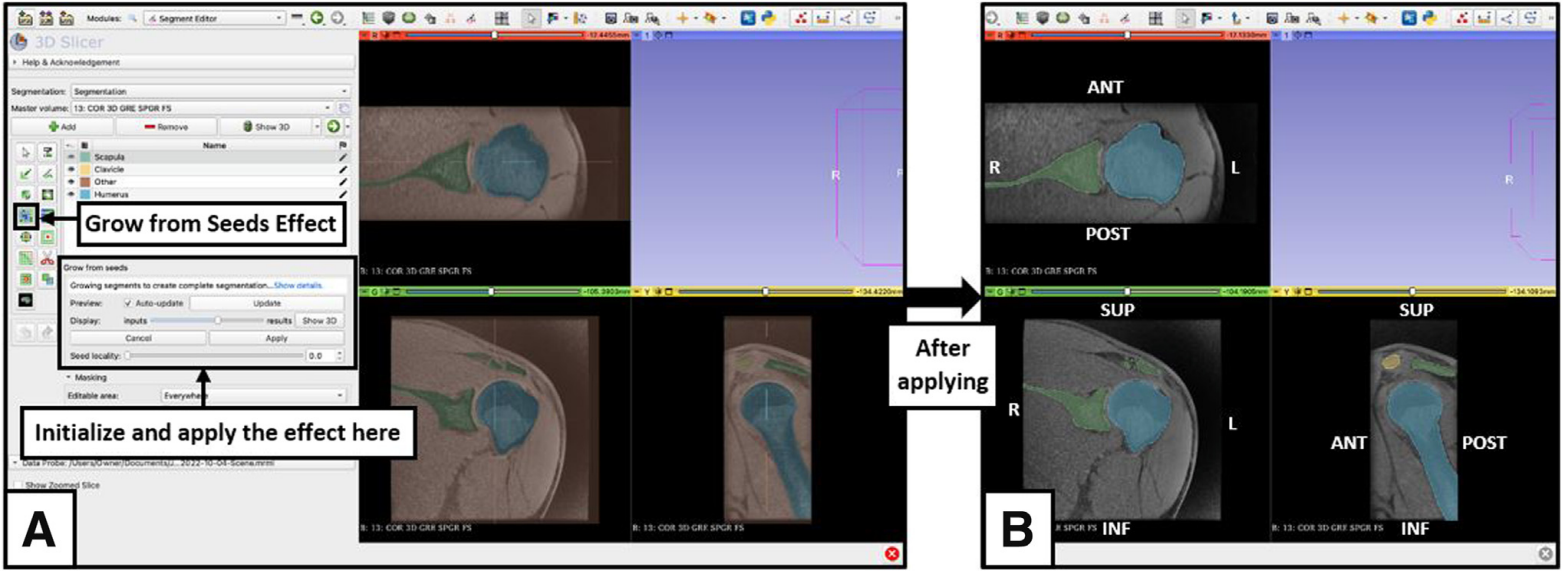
Use of the ‘grow from seeds’ effect from the Segment Editor module view of the 3D Slicer application. (A) Preview; (B) Applied. The ‘Grow from Seeds’ effect allows the computer software to analyze the painted sections of the scan, extrapolate, and apply the colors of different segments to areas of similar intensity, based on location, size, and shape. In a scan of good quality, this will separate the structures from each other and show the segments colored in the entire scan. Ideally, the segments should be outlined by the appropriate color; however, quality, contrast, and magnetic resonance imaging data set sequencing may impact the validity of the segmentation. (ANT, anterior; INF, inferior; L, left; POST, posterior; R, right; SUP, superior.)
